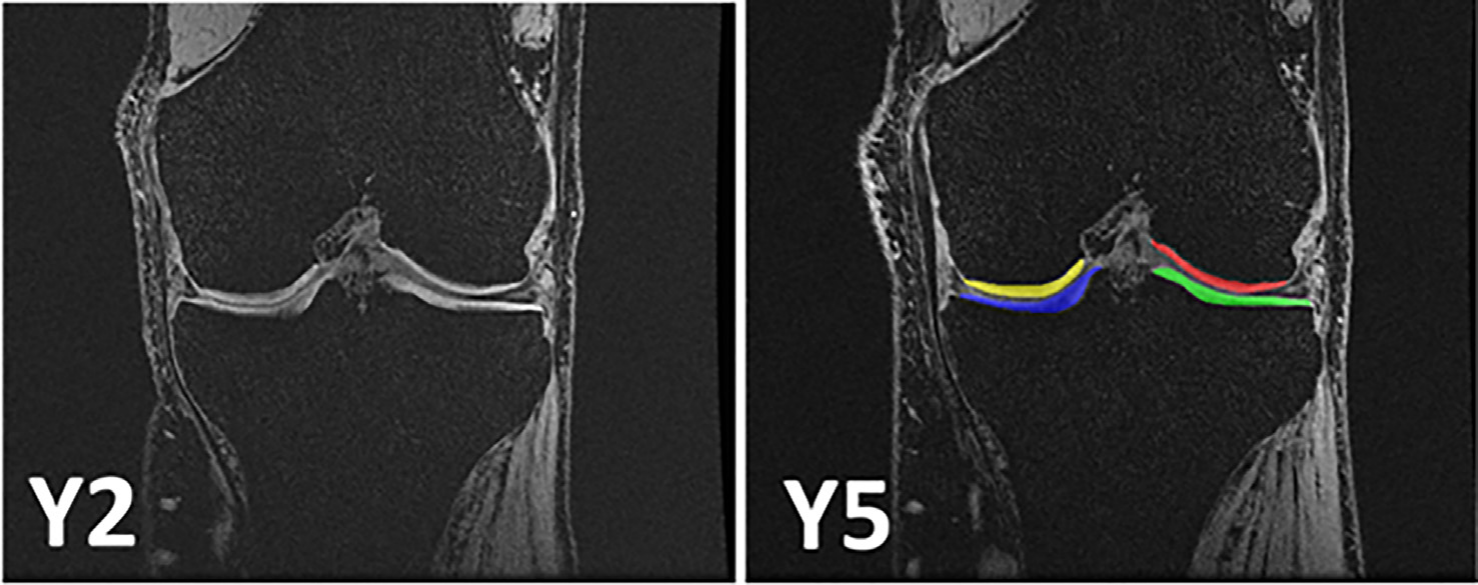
Coronal fast low angle shot (FLASHwe) MRI acquired over the post-treatent period of the FORWARD study at year 2 (Y2) and year 5 (Y5). The last sprifemin treatment (i.a. injection) was applied at 18 months (i.e. 6 months prior to Y2). Y2 image without segmentation and Y5 image with segmentation of the weight-bearing femorotibial joint cartilages. Blue ​= ​medial tibia; yellow ​= ​medial femur; ​= ​MFTC; Green ​= ​lateral tibia; red ​= ​lateral femur; ​= ​LFTC
